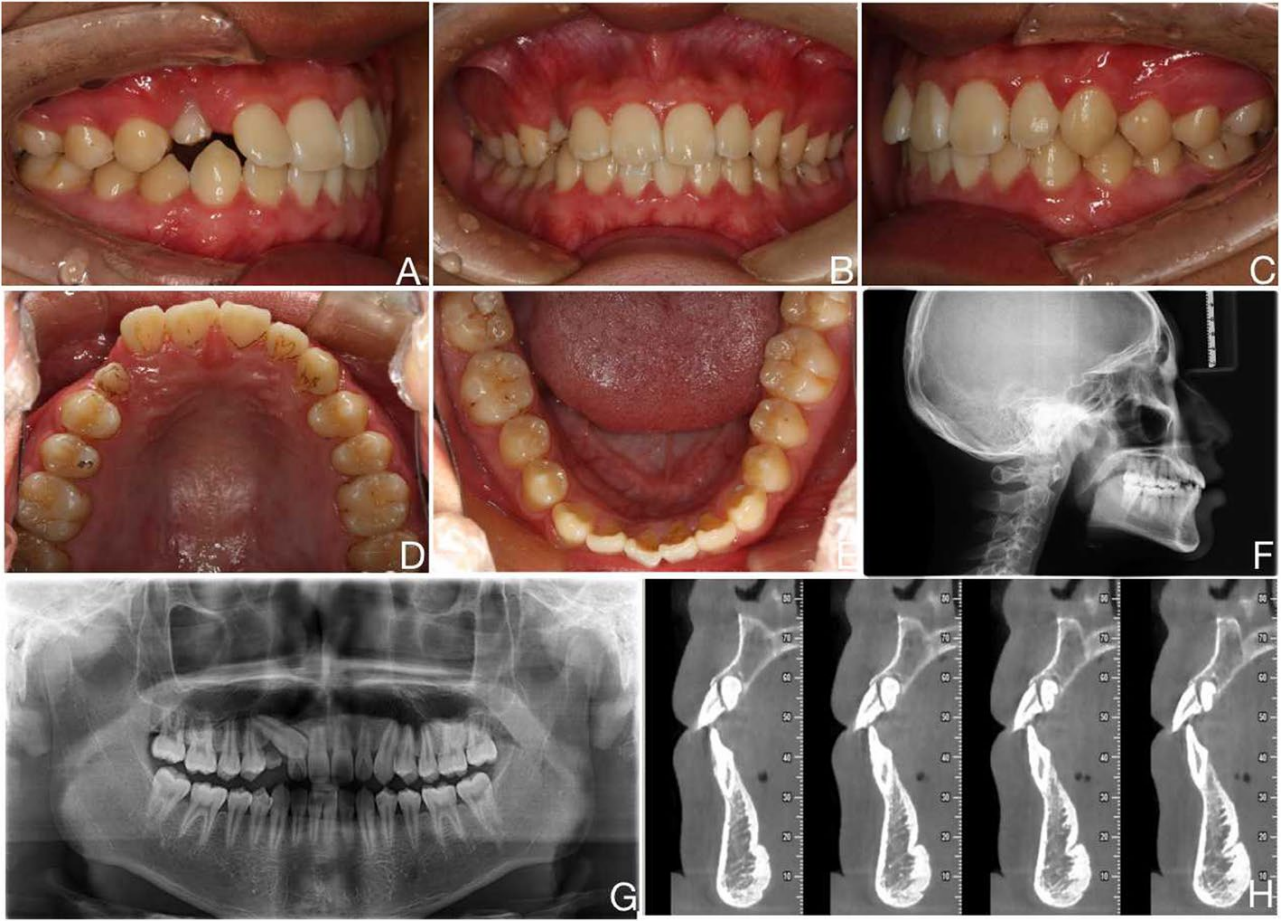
Pre-treatment oral photograph and radiograph
